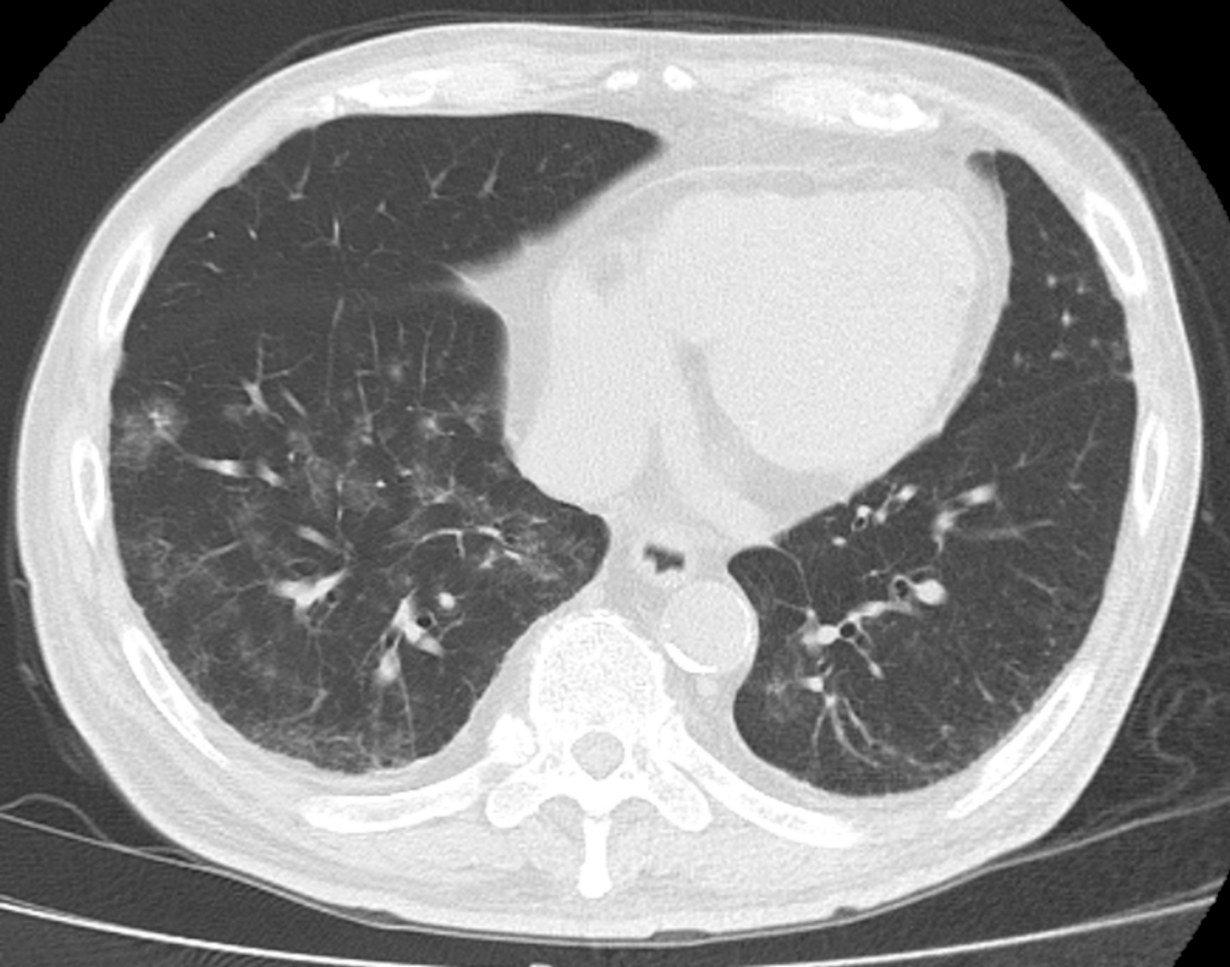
Pre-treatment non-contrast CT scan of the chest A non-contrast CT scan of the chest showing ground-glass opacities along the airways of the right lower lobe. Minimal bilateral pleural effusion is noted, with no evidence of mediastinal lymphadenopathy. CT: computed tomography
